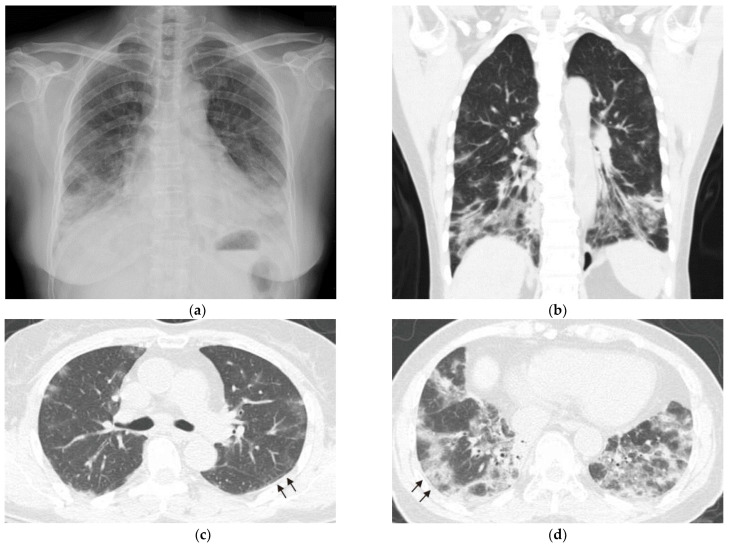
Chest imaging at initial presentation (a) Chest radiograph revealed reticular opacities and consolidations in bilateral lower lungs. (b–d) Computed tomography scans revealed subpleural sparing patterns (arrows) and multifocal ground-glass opacities in bilateral basal distribution, indicating nonspecific interstitial pneumonia.
